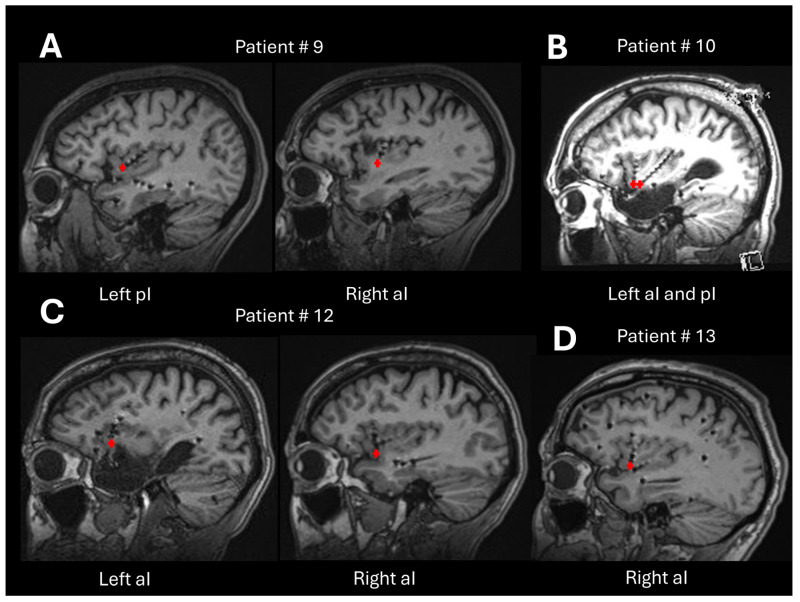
(A–D) Post-implantation sagittal MRI slices from the patients enrolled in the study (patients #9, #10, #12, and #13). Red crosses indicate the exact locations of the microcontacts of the hybrid electrodes. In all cases, the microcontacts are located within the antero-inferior portion of the insular cortex.
